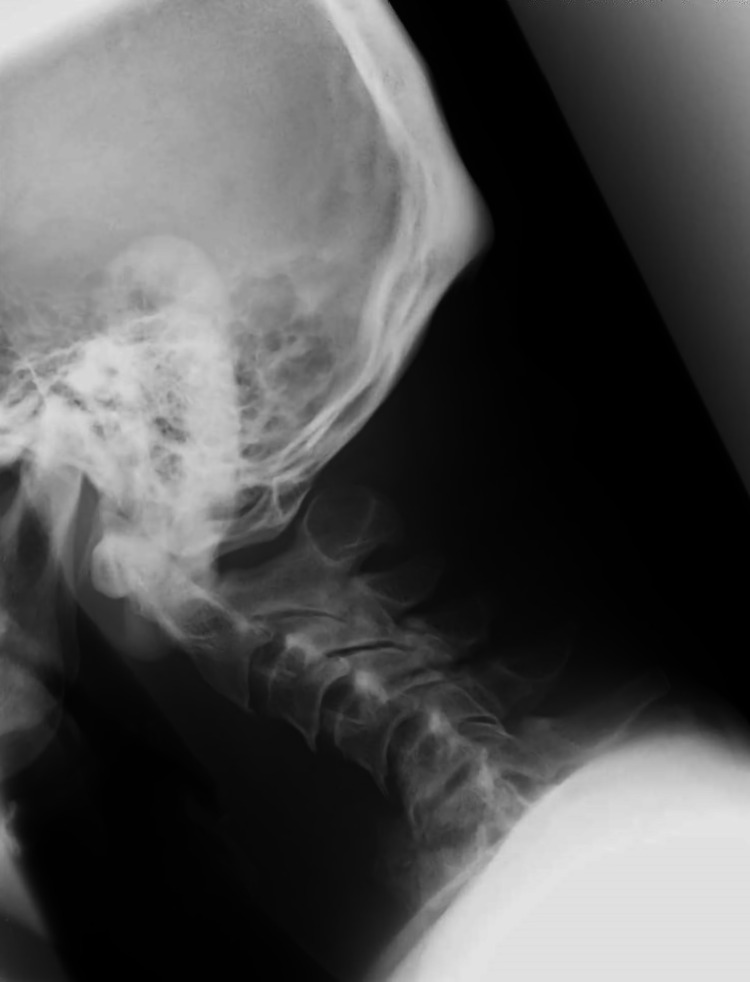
Preoperative cervical radiography Lateral view of the cervical radiograph on the admission day revealed severe destruction of the vertebral bodies and kyphotic changes at the C5–C7 vertebral levels.
